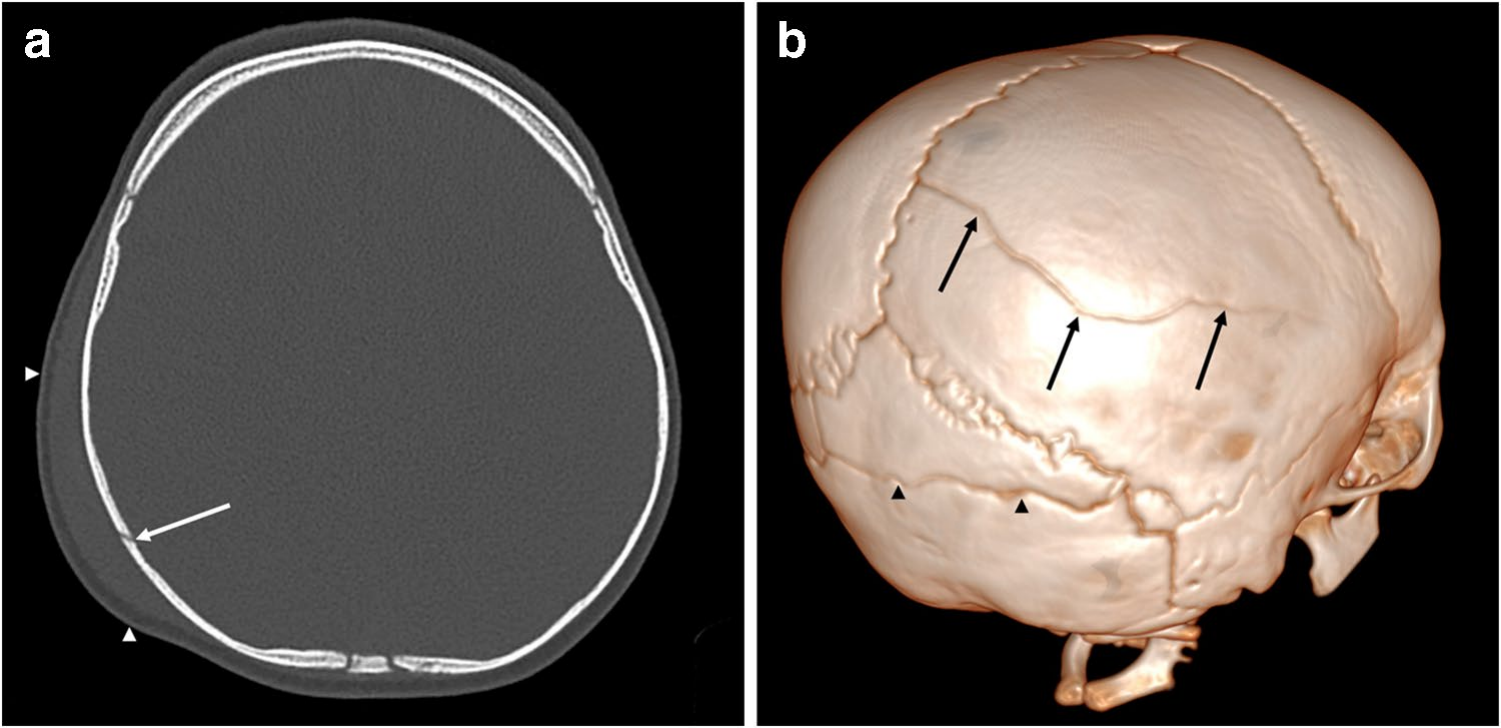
Head CT imaging in a 9-month-old male. a The selected axial slice (bone window) demonstrates a right parietal skull fracture (*arrow*) with overlying soft tissue swelling (*arrowheads*). b The 3D volume-rendered reconstruction (right posterior oblique view) from (a) also demonstrates the right parietal skull fracture (*arrows*). Note the accessory suture in the occipital bone (*arrowheads*) which is a normal anatomical variant alongside several intrasutural bones in both lambdoid sutures. This accessory suture can be differentiated from a fracture due to its ‘zigzag’ morphology, sclerotic margins (on multiplanar imaging) and regular interdigitations without overlying soft tissue swelling. Moreover, accessory sutures are commonly located in the occipital bone
